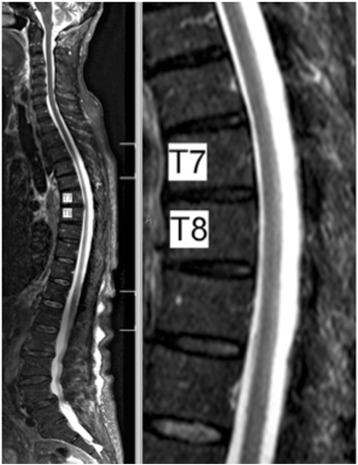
MRI Scan sagittal total-spine MRI scan after the event showing no sign of myelopathy
